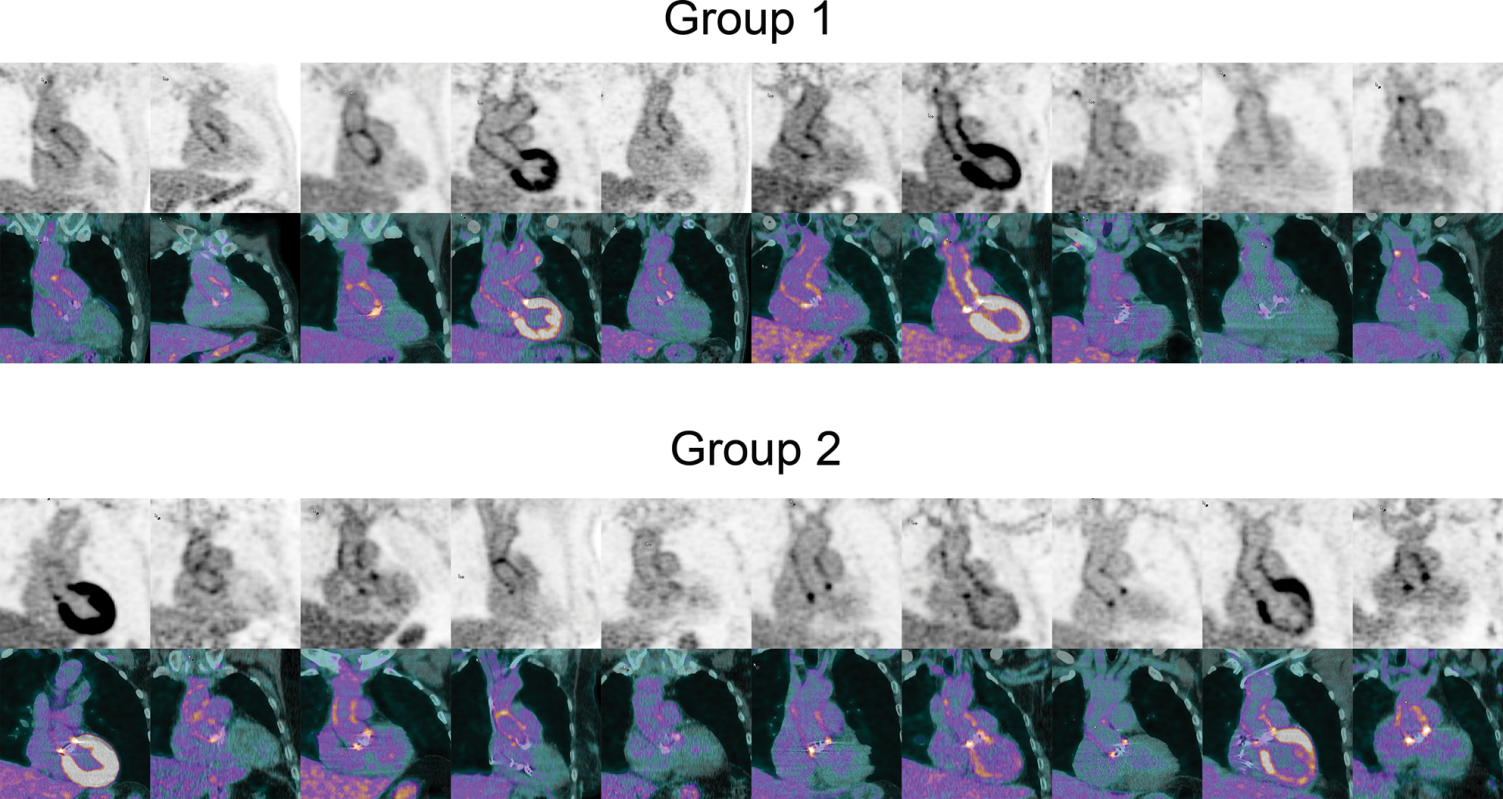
^18^F-FDG uptake around the prosthesis on coronal views of attenuation-corrected (AC) images and fused attenuation-corrected images with CT in all patients. Scaling was set the same for all AC images and represents SUV with a range of 0-7
